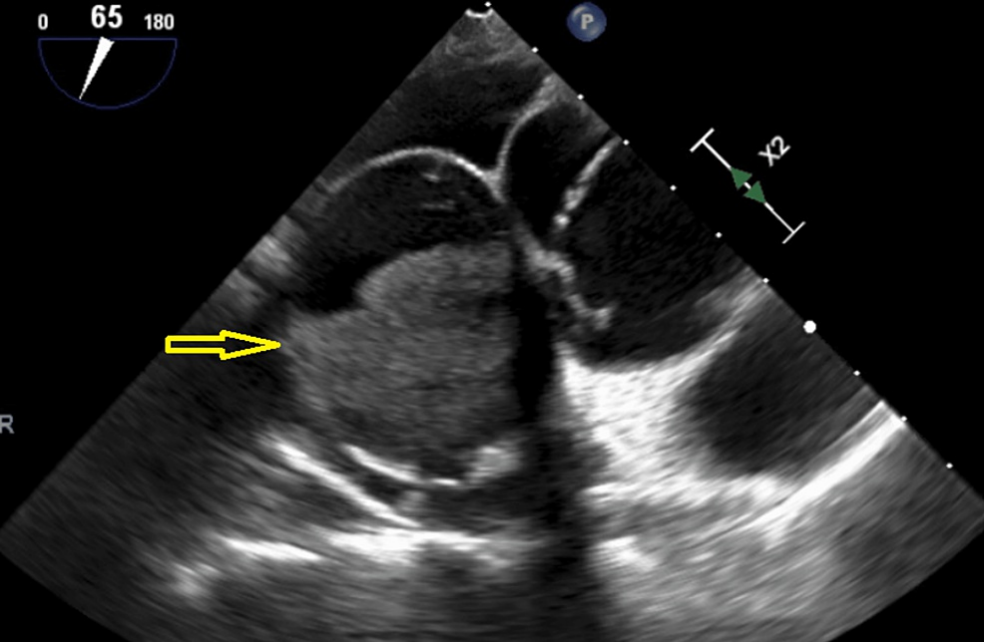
Transthoracic echocardiogram. Yellow arrow showing right atrial mass.
